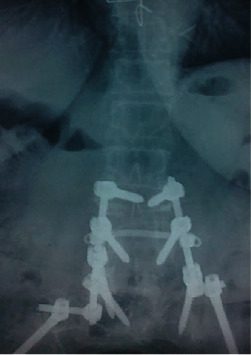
Postoperative anteroposterior roentgenogram of the lumbar spine.
